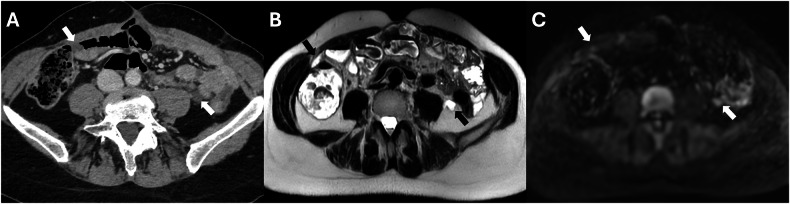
Benign peritoneum mesothelioma in a 61-year-old woman. A CT porta-venous phase, axial plane showed cystic implants in the right and left iliac fossa (white arrows), confirmed in MRI T2w (B, black arrows) and DWI (b = 800 mm^2^/s) (C, white arrows)Micronodular pattern: indicating the presence of peritoneal implants less than 5 mm in diameter, affecting the peritoneum and the mesenteric fat (Fig. 3). Infiltration of the small bowel mesentery may produce characteristic stellate patterns, which consist of increased mesenteric fat attenuation and perivascular soft-tissue thickening caused by the infiltration of the perivascular bundles [26].
